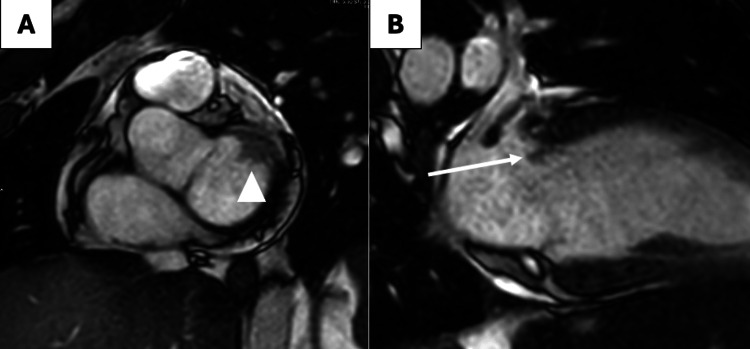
Cardiac MRI in short axis (A) and two-chamber (B) planes showing soft tissue structure attached to the mitral annulus at the atrial side (arrowhead) with a small mobile floating portion (arrow).
